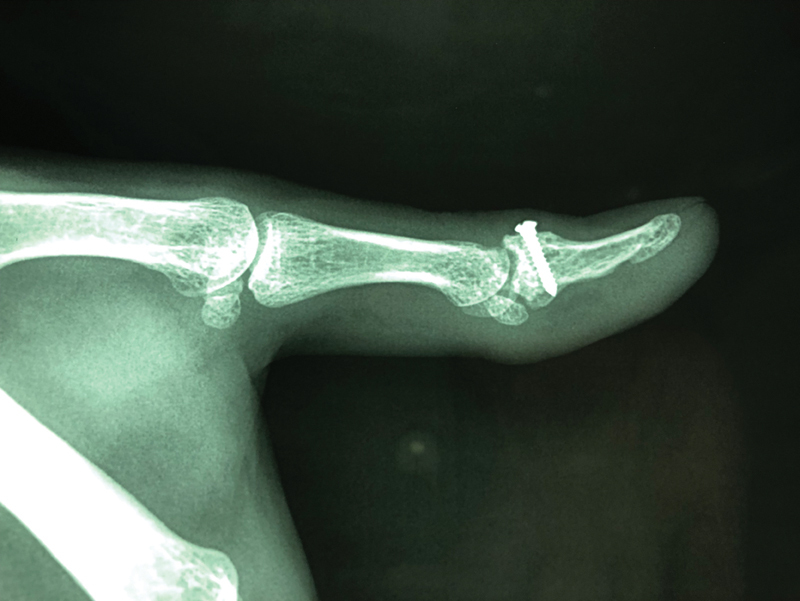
Eighteen months postoperative lateral radiograph demonstrating the full extension of the thumb's interphalangeal joint. There was no gap at the articular surface. All the bone fragments were consolidated.
